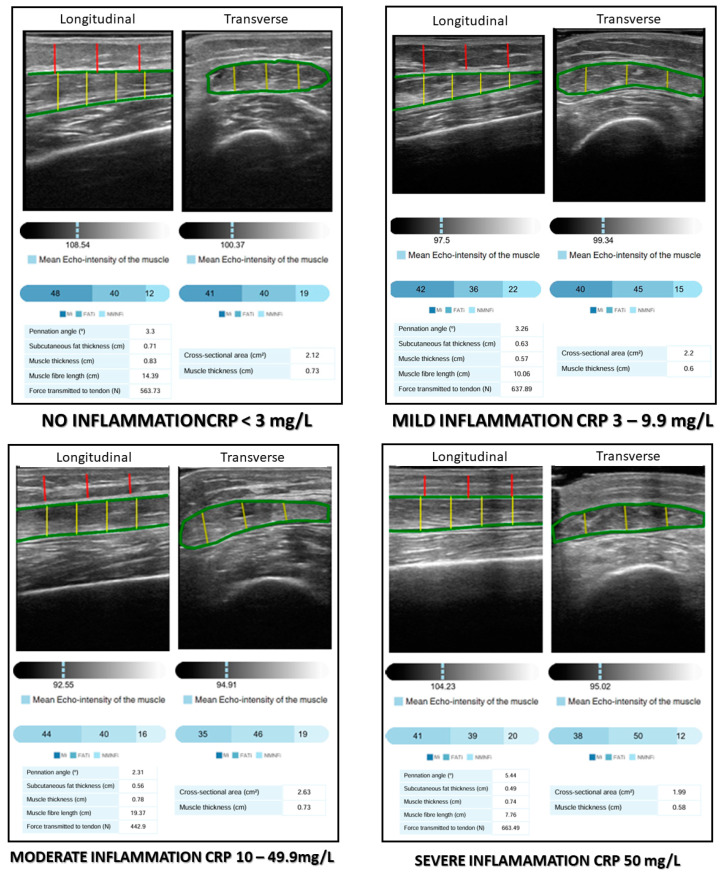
AI-assisted rectus femoris ultrasonography and degrees of inflammation. MiT: muscle index; FATiT: fat index; NMNFiT: no muscle no fat index; CRP: C-reactive protein.
